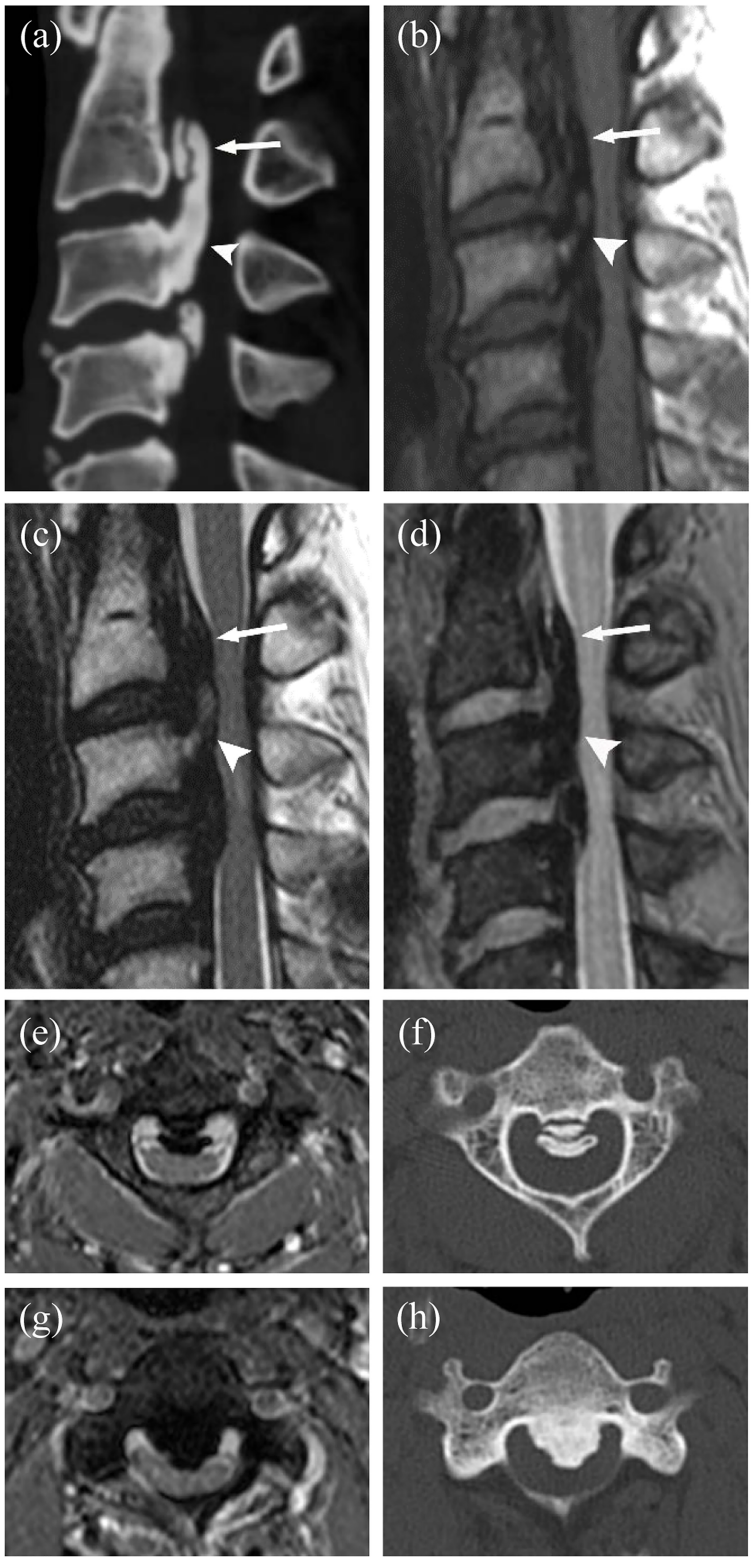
A 42-year-old man with cervical OPLL and DO. (a) Sagittal CT image of the cervical spine, showing OPLL with a double-layer DO (arrow) at the C2 body and a single-layer DO (arrowhead) at the C2-3 disc level through the C4 body level. (b) Sagittal T1WI showing various signals of OPLL. DO at the C2 level (arrow) was correctly diagnosed by both readers; however, one reader incorrectly interpreted this signal as a single-layer sign. The DO of the C2-3 disc level through C4 body level (arrowhead) was correctly interpreted as a single-layer sign by both readers. (c) Sagittal T2WI showing various signals of OPLL. The presence of DO at the C2 level (arrow) was correctly diagnosed by both readers; however, both incorrectly interpreted this signal as a single-layer sign. The DO of the C2-3 disc level through C4 body level (arrowhead) was correctly interpreted as a single-layer sign by both readers. (d) Sagittal mFFE image showing a double-layer sign (arrow) and a single-layer sign (arrowhead), which were correctly interpreted as signs of a double-layer and a single layer DO, respectively, by both readers. (e, f) Axial mFFE (E) and axial CT (F) images at the C2 body level (arrow, a-d) showing a double layer sign. (g, h) Axial mFFE (G) and axial CT (H) images obtained at the C3 body level (arrowhead, a-d) showing a single layer sign.
